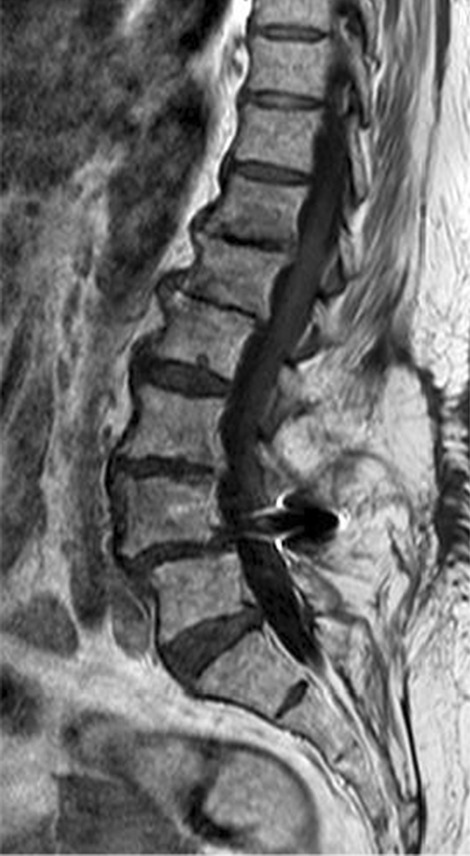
Sagittal T1 injected magnetic resonance imaging
